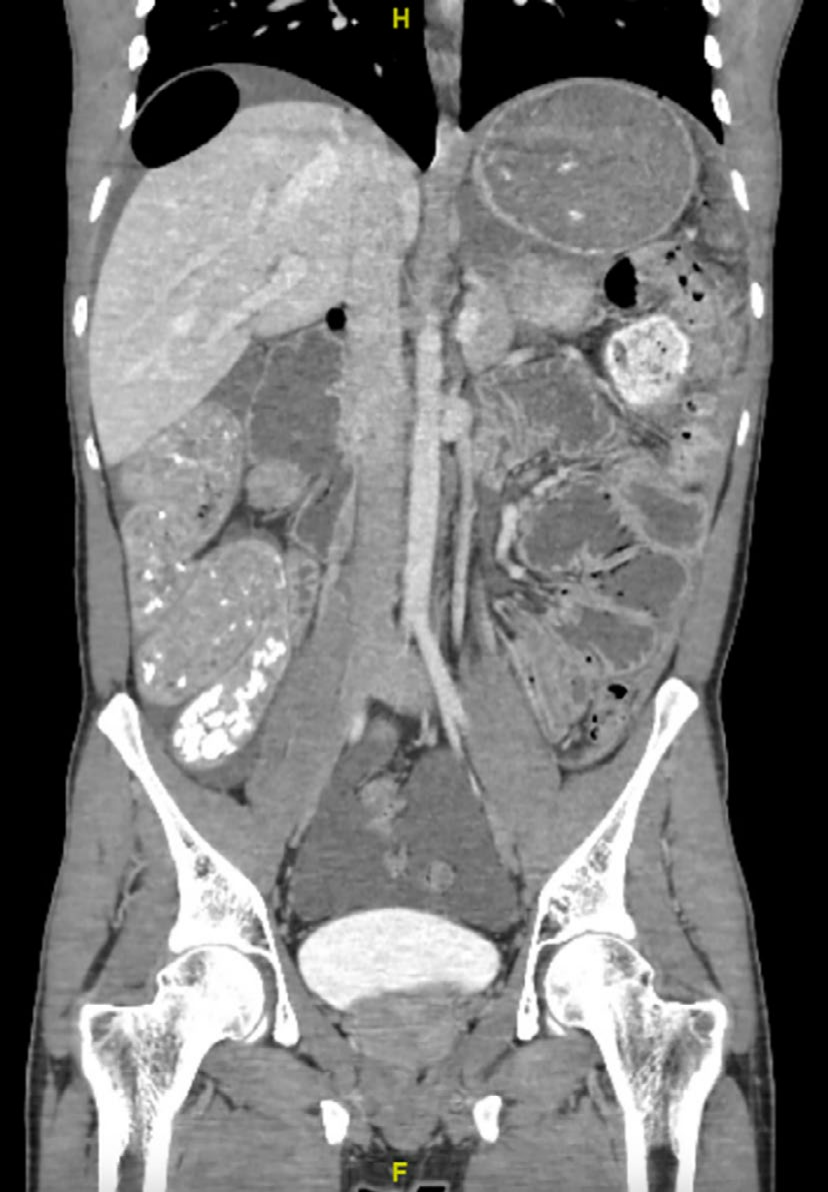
Coronal cross‐section of CT abdomen and pelvis with diffuse fluid‐filled loops of bowel and a focal section of air underneath the diaphragm in the right upper quadrant. H: toward patient head and F: toward patient feet.
